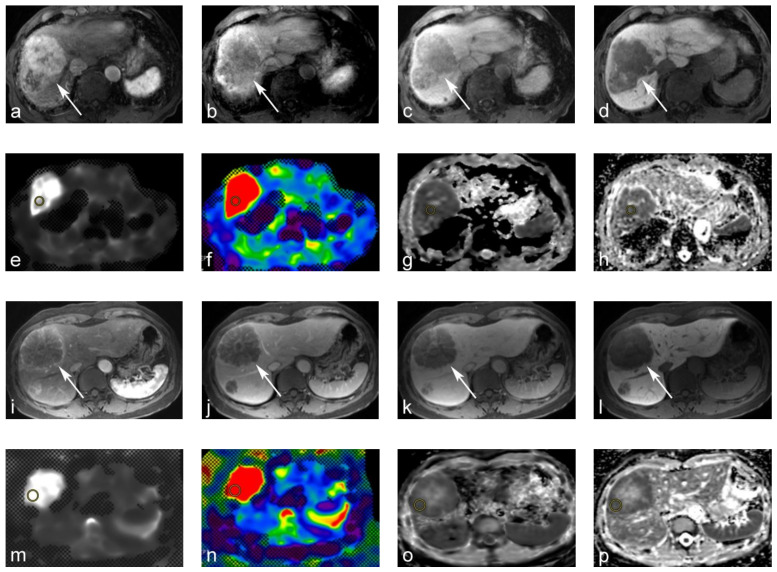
69-year-old male with moderately differentiated hepatocellular carcinoma (HCC). (a–d) Gd-EOB-DTPA-enhanced magnetic resonance image (MRI): (a) tumors show arterial hyperenhancement on the arterial-phase. (b,c) Tumor shows a washout on the portal venous and transitional phase; (d) tumor shows a hypointensity on the hepatobiliary phase. (e,f) MR elastography (MRE). The tumor yielded a stiffness of 10.71 kPa on the MRE. (g,h) Apparent diffusion coefficient (ADC) maps. The tumor yielded a value of 0.63 × 10^−3^ mm^2^/s on the shifted ADC (sADC) map, and a value of 0.93 × 10^−3^ mm^2^/s on the ADC map. 60-year-old male with metastatic hepatic cancer from colorectal cancer. (i–l) Gd-EOB-DTPA-enhanced MRI. (i,j) Tumor showed rim-like enhancements on the arterial and portal venous phase. (k,l) The tumor was hypointense on the transitional and hepatobiliary phases. (m,n) MRE. The tumor yielded a stiffness of 15.59 kPa on MRE. (o,p) ADC maps. The tumor also yielded an sADC value of 0.72 × 10^−3^ mm^2^/s on the sADC map, and 0.86 × 10^−3^ mm^2^/s on the ADC map.
